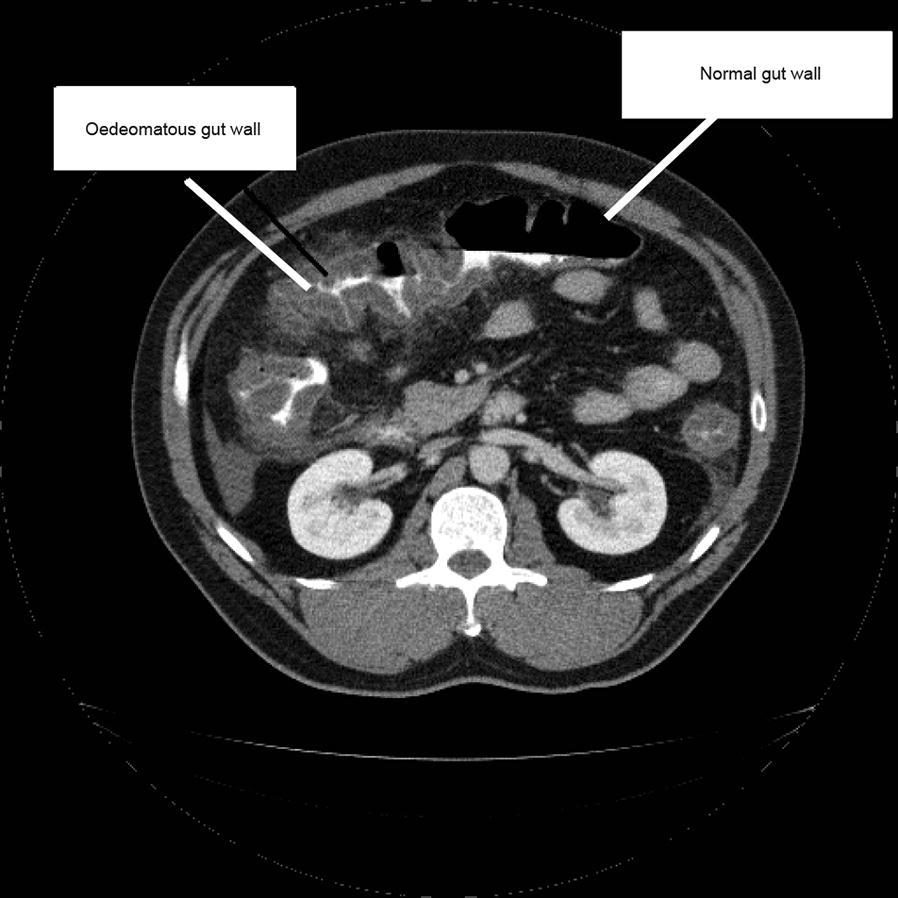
Abdominal computed tomography image obtained at admission of a 47-year-old male patient with HAE type I with abdominal angioedema
